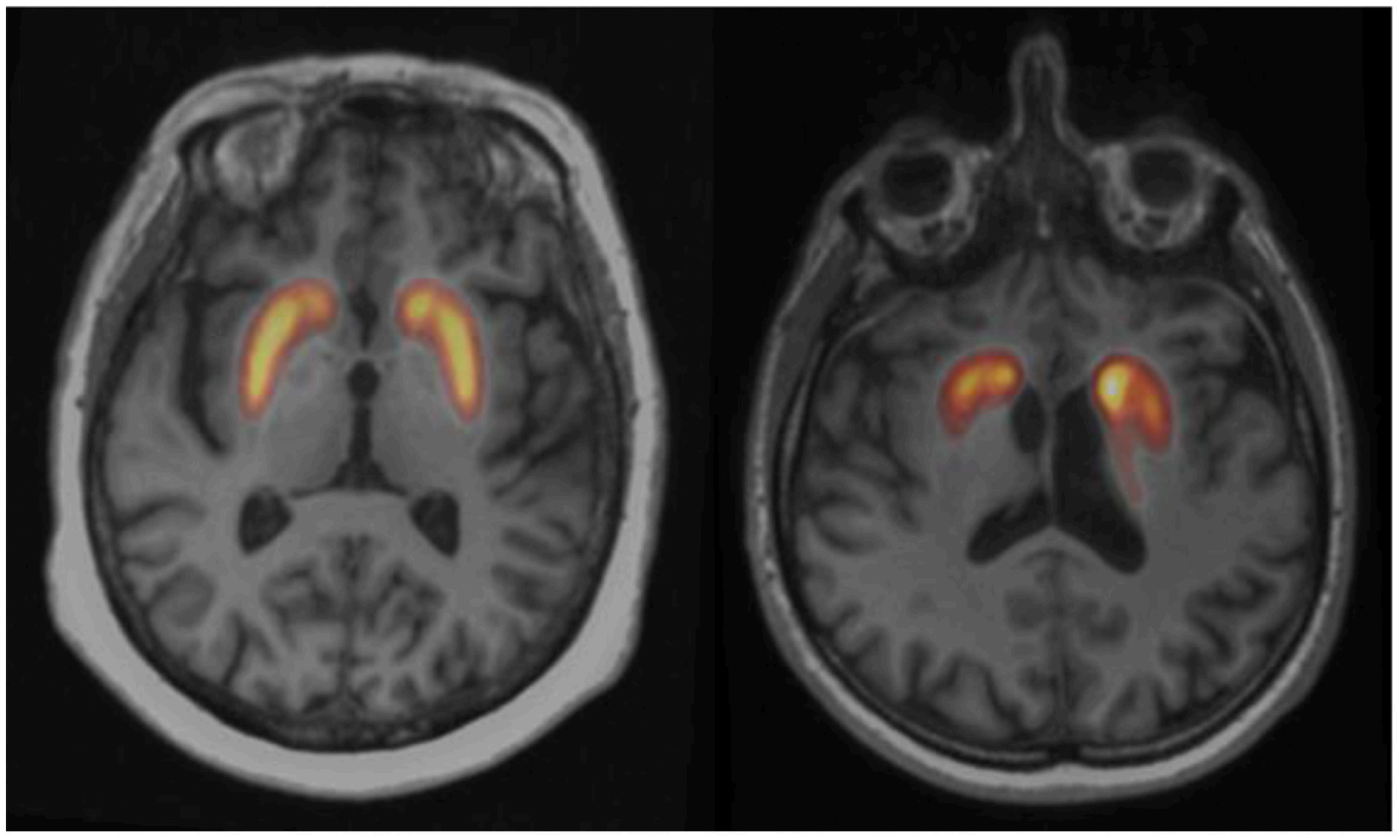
Fusion axial slices between PET and MRI of the [^18^F]LBT-999 uptake at the level of the striatum in a control subject (left) and a drug-naïve patient with early Parkinson disease (right). The radiopharmaceutical uptake is asymmetrically decreased in Parkinson patient.
